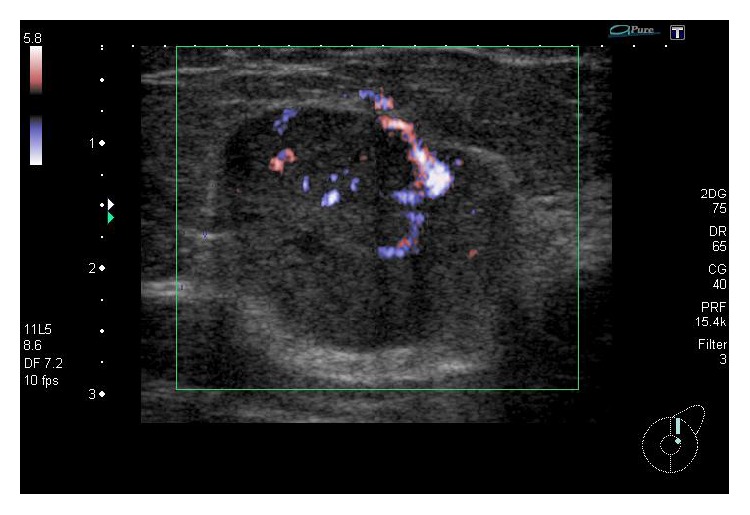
A breast ultrasonography image shows well-demarcated, highly abundant blood flow and a cystic lesion. These findings suggest a breast tumor with malignancy.
